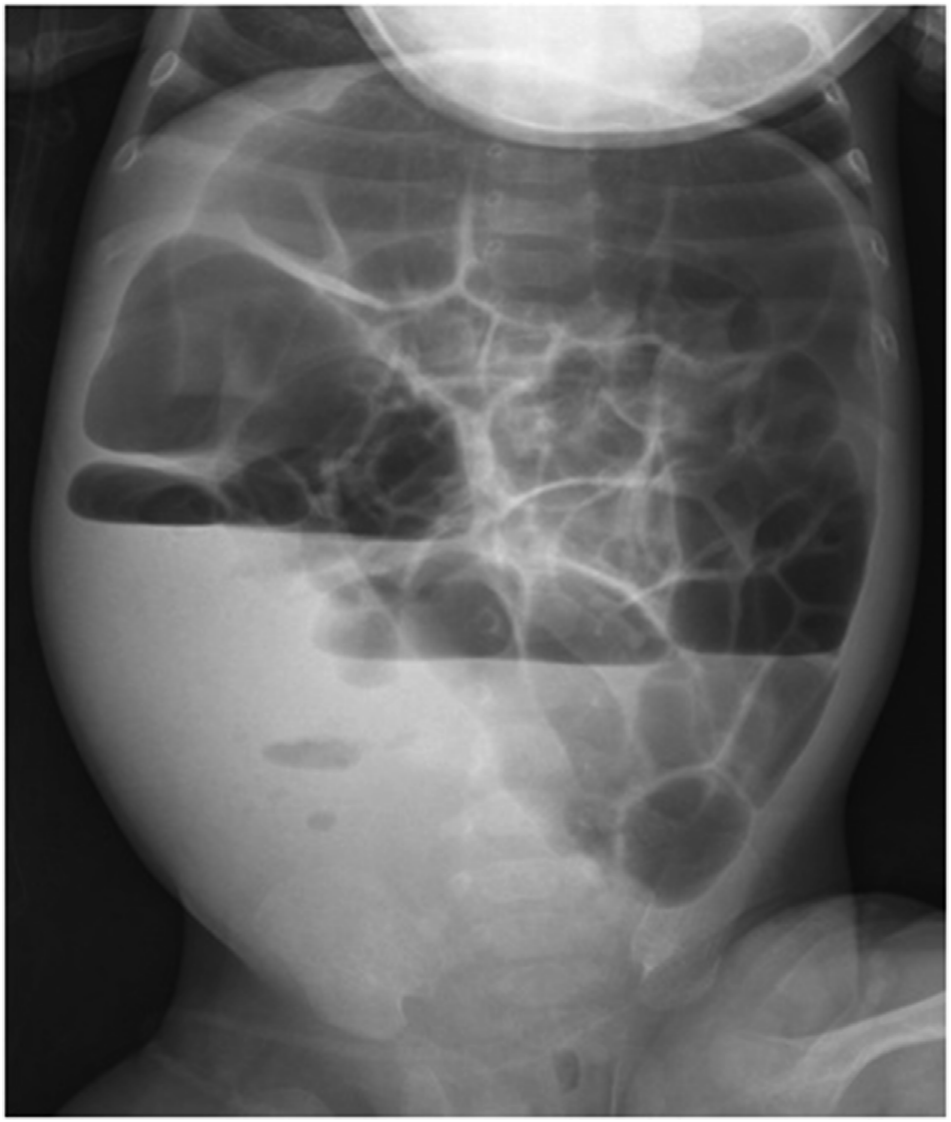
Abdominal radiograph showing features of intestinal obstruction with multiple air fluid levels. A soft tissue density is seen in right iliac and lumbar regions displacing bowel loops to left side. Both small and large bowel loops are dilated.
